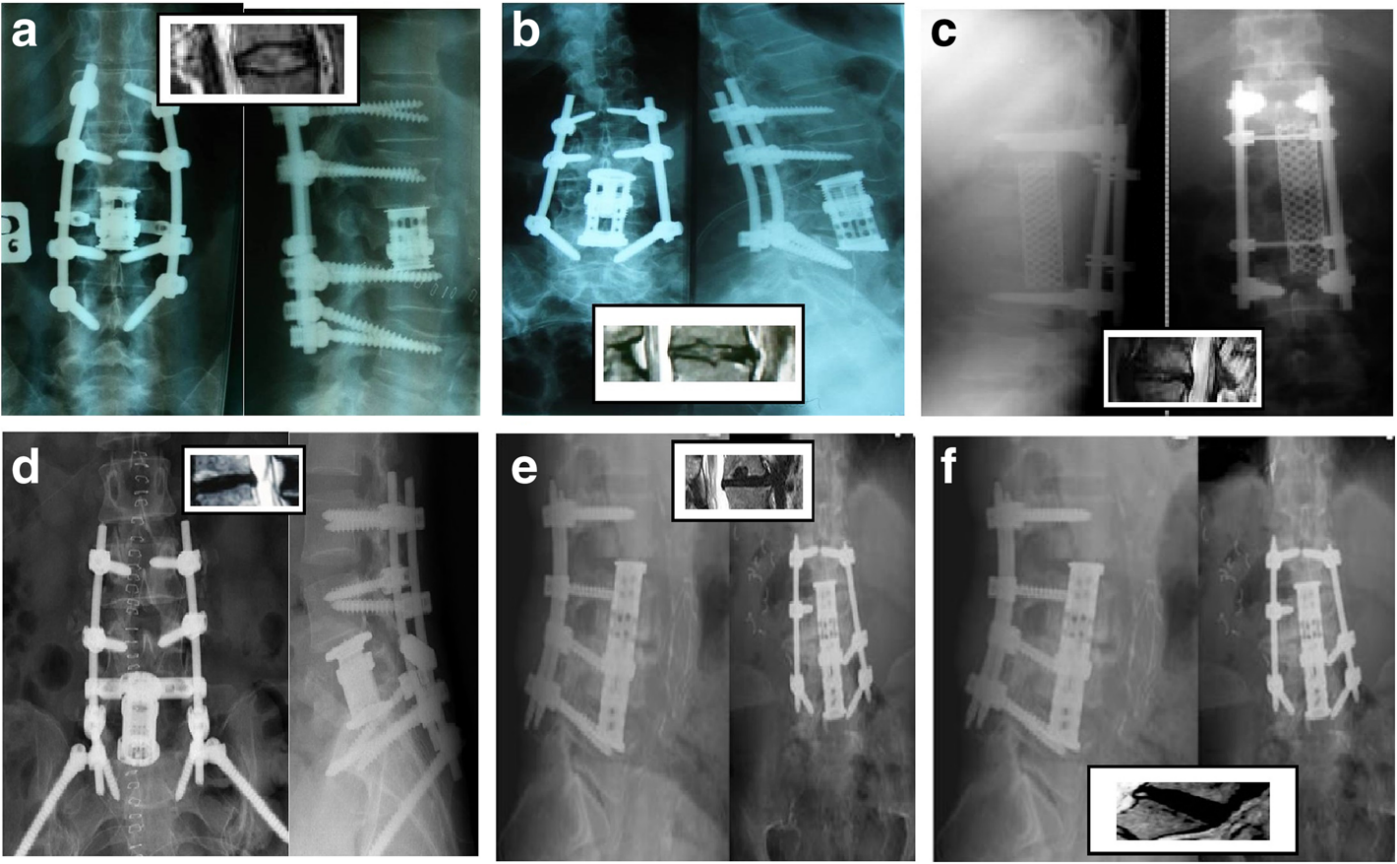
a–f Examples of six postoperative radiograms and MRI scans focused on different types of ASD
